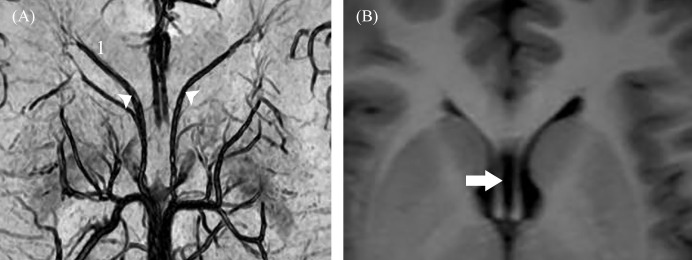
A, Transverse SW image showing the anterior septal vein and septal points. The distance between bilateral septal points (white arrowhead) is 6.6 mm. B, Transverse T1-weighted image from the same volunteer showing a wider cavum septi pellucidi. (1, anterior septal vein; white arrow, cavum septi pellucidi).
